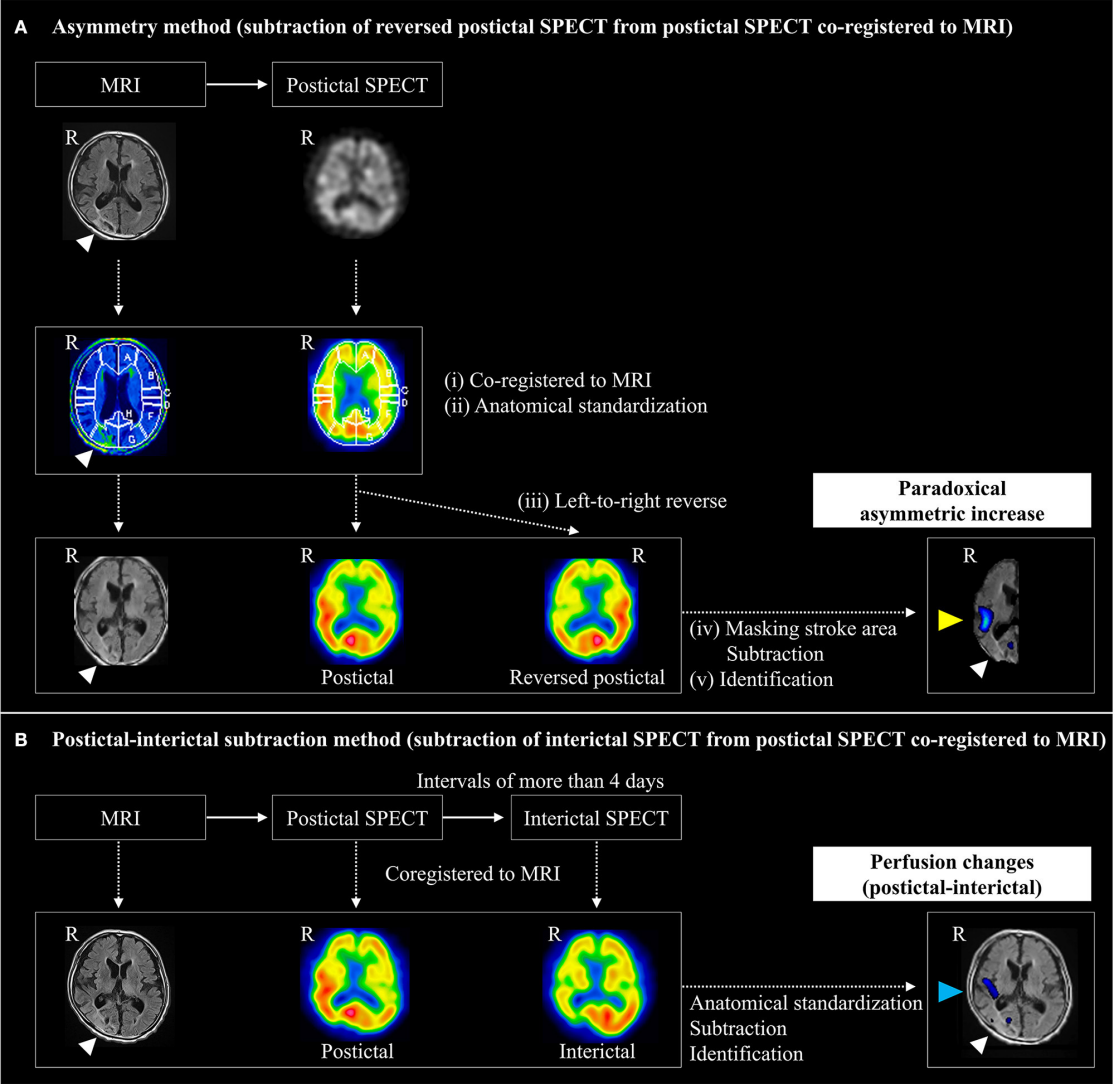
Imaging analysis protocol for detecting prolonged postictal hyperperfusion. Postictal hyperperfusion was evaluated by the following two methods: (A) the asymmetry method and (B) the postictal-subtraction method. (A) The asymmetry method was devised for detecting a higher perfusion area (yellow arrowhead) in the hemisphere ipsilateral and adjacent to stroke lesions (white arrowhead), compared to the corresponding contralateral area, which was defined as a paradoxical asymmetric increase by subtracting anatomically standardized non-reversed SPECT imager from left-to-right reversed postictal SPECT images to obtain non-reversed – reversed difference. (B) The postictal-interictal method was devised based on detecting an area of perfusion postictal-interictal changes (arrowed in blue): anatomical standardized postictal and interictal SPECT images were subtracted to obtain the postictal – interictal difference. R, right.
